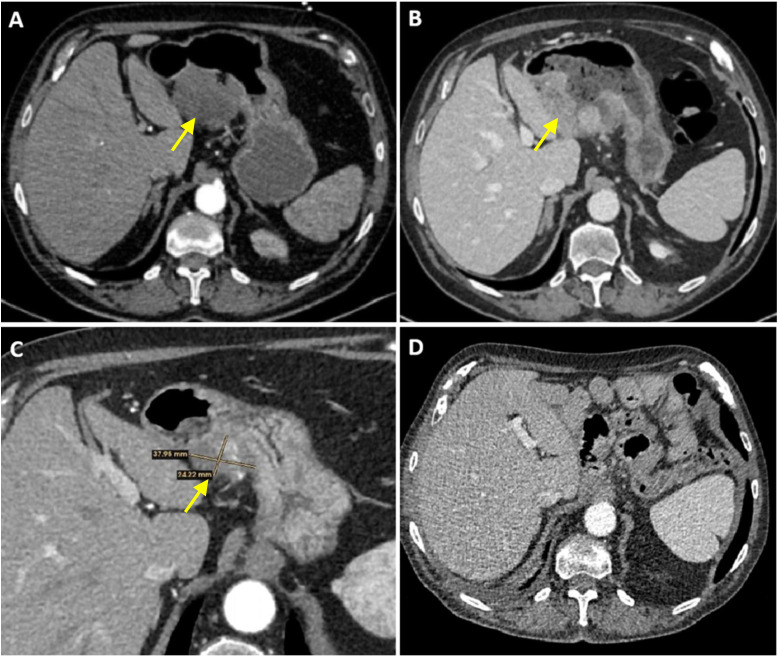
(A) Abdominal CT scan at diagnosis, describing a prominent exophytic mural lesion on the mesenteric border of the gastric antrum. (B) Abdominal CT scan in September 2024 revealing a loco-regional progression despite 4 cycles of FLOT chemotherapy. (C) Abdominal CT scan in December 2024 shows a partial tumor response after seven cycles of nivolumab monotherapy. (D) Abdominal CT scan in December 2025 shows no evidence of disease after salvage surgery.
